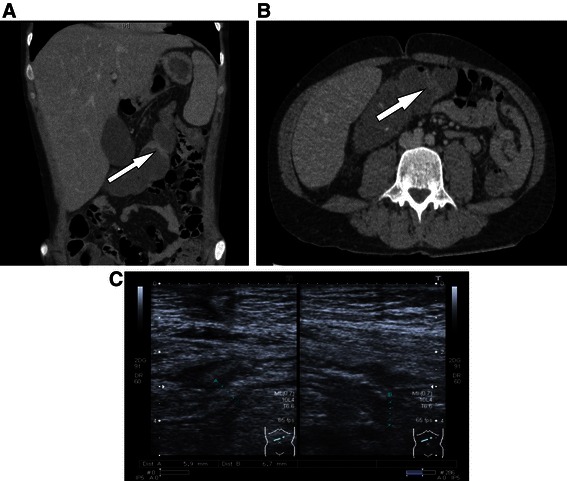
Radiographic pictures of the stenosis. 1A and 1B: CT-scan of the stenosis of the transverse colon (1A: frontal view; 1B: horizontal view). 1C: Ultrasound picture of the stenosis. Stenosis was 5.9 mm × 6.7 mm in diameter. The intestinal wall appeared thickened and hypoechoic. There was no intra- or extra-mural vascularization present consistent with level I according to the Limberg classification.
